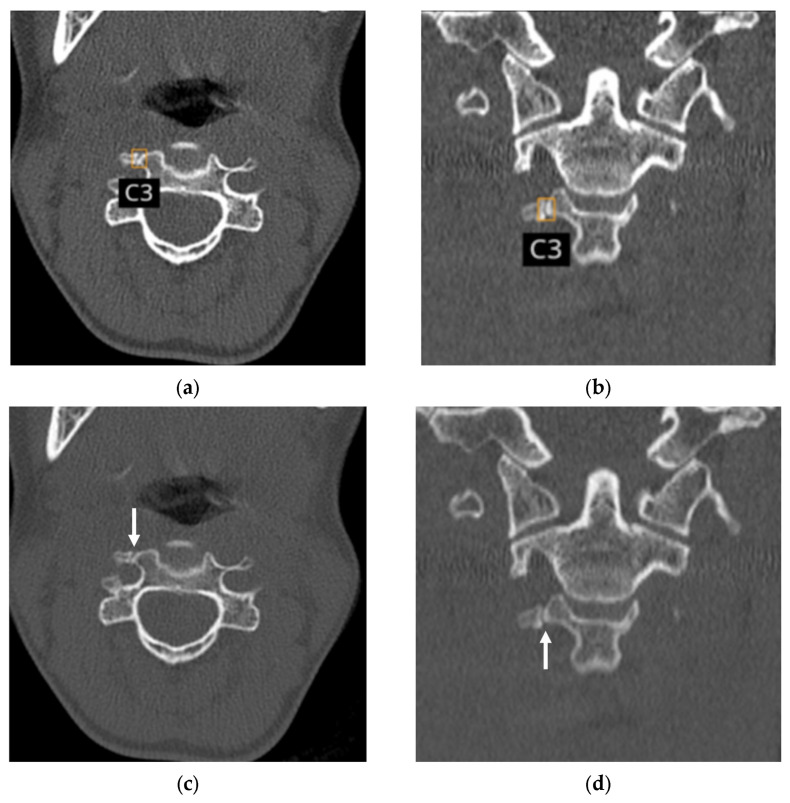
Non-enhanced CT images in a 27-year-old female with a non-acute fracture of the right transverse process of C3. The first radiologist classified the case as “negative”, whereas the second radiologist labeled the non-acute fracture as “positive”, representing a discrepant case between the first two radiologists. The third radiologist classified the case as “negative”. (a,b) The AI application falsely localized the non-acute fracture, misinterpreting it as acute. The spinal level was correctly labeled. (c,d) Corresponding axial (c) and coronal (d) CT images without bounding boxes demonstrate the non-acute fracture of the right C3 transverse process, which is smooth and corticated (arrows).
